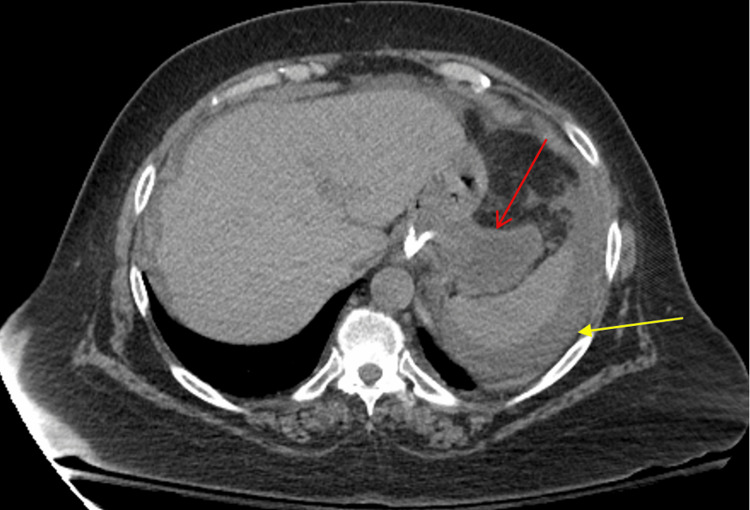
Repeat CT abdomen and pelvis with IV contrast (axial view) performed after an acute change in abdominal pain demonstrates a decompressed gastric remnant (red arrow) with free fluid (yellow arrow) concerning a perforation of the excluded stomach
